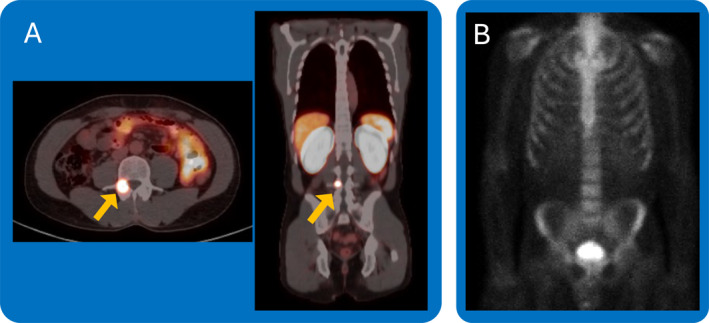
Discordant findings on PSMA PET versus technetium‐99m bone scan in a patient with de novo metastatic hormone sensitive prostate cancer. (A) Single bone lesion at the right L4 pedicle was PSMA‐avid on axial (left) and coronal (right) views. (B) Techneitum‐99m bone scan without uptake in the right L4 pedicle. How do you manage these discordant imaging findings? PET indicates positron emission tomography; PSMA, prostate‐specific membrane antigen.
